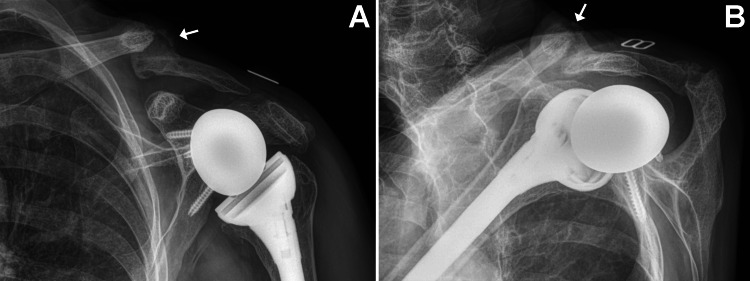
Radiographs taken 13 months after RTSA. Radiographs of the left shoulder taken 13 months after the RTSA showing evidence of healing of the acromion fracture and signs of healing at the site of the clavicle fracture, which had become 100% displaced (arrows). There was mild pain with palpation of the clavicle fracture site, but no pain with palpation of the acromion fracture site. (A) Anterior-posterior view and (B) scapula Y view. RTSA: Reverse total shoulder arthroplasty
